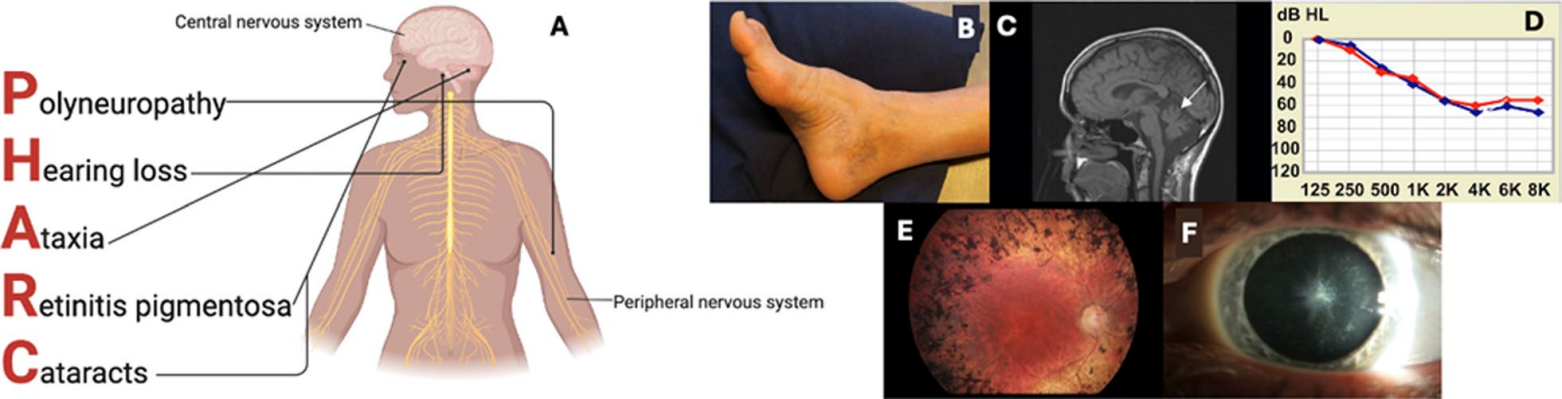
Main symptoms of the PHARC syndrome with observed findings from known PHARC patients. A An overview of all clinical symptoms of PHARC syndrome linked to their organ system. B Pes cavus and hammer toes can be mild signs of peripheral neuropathy. C MRI scan showing cerebellar atrophy (white arrow). D Audiogram showing sensorineural hearing loss (SNHL) of both right (red curve) and left (blue curve) ear. E Fundus showing bone-spicule-shaped pigment deposits in the mid-periphery, pallor of the optic disc, attenuation of retinal vessels and maculopathy. F Star-shaped cataract of the posterior pole of the lens
